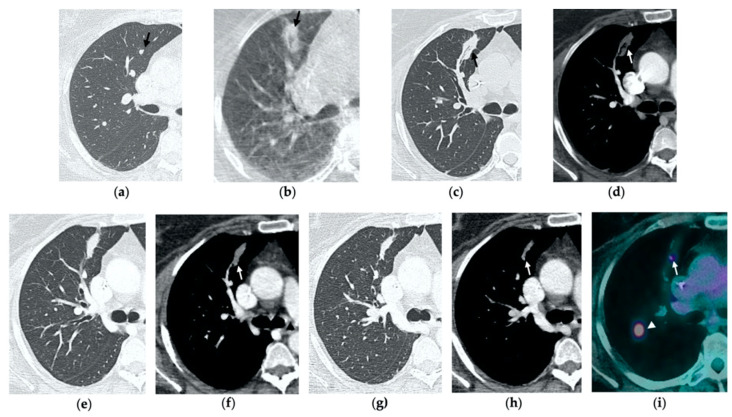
63-year-old-woman (same patient in Figure 3) with pulmonary metastasis from CRC in the right upper lobe. (a) Axial CT before treatment (black arrow). (b) Cone-beam CT image obtained at the end of the procedure shows GGO (black arrow) around the treated lesion. (c,d) Axial 2-month follow-up CT images show an elongated consolidation with hypoattenuating bubbles (black arrow) and no contrast uptake (white arrow). (e,f) Axial 5-month follow-up CT images demonstrate a tiny nodular uptake of contrast on the posterior margin of the consolidation (white arrow), suggestive of residual disease. (g,h) On axial CT images after 8 months the nodular enhancement persists (white arrow). (i) PET/CT image at 9 months proves residual disease on the treated lesion (white arrow) as well as simultaneous metastasis (white arrowhead) in the posterior segment.
